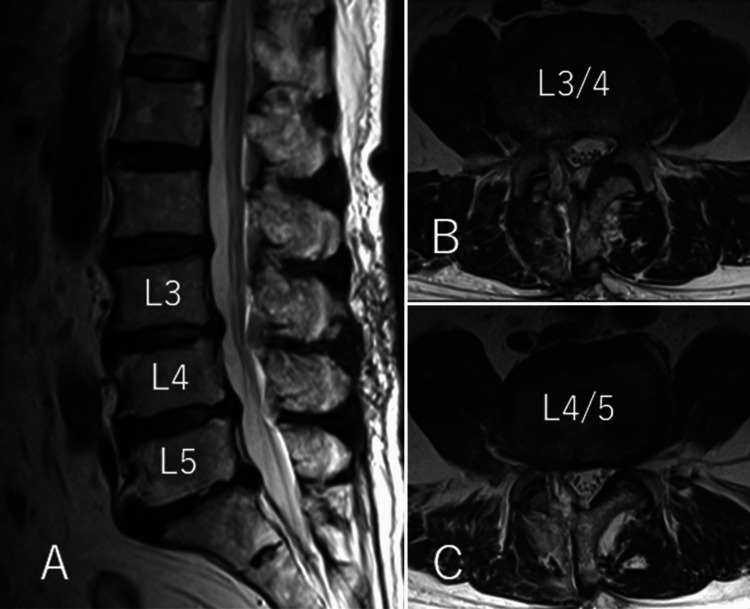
Postoperative MRI (A) Sagittal. (B,C) Axial. T2-weighted MRI performed postoperatively after 10 days showing good decompression in the spinal canal.
